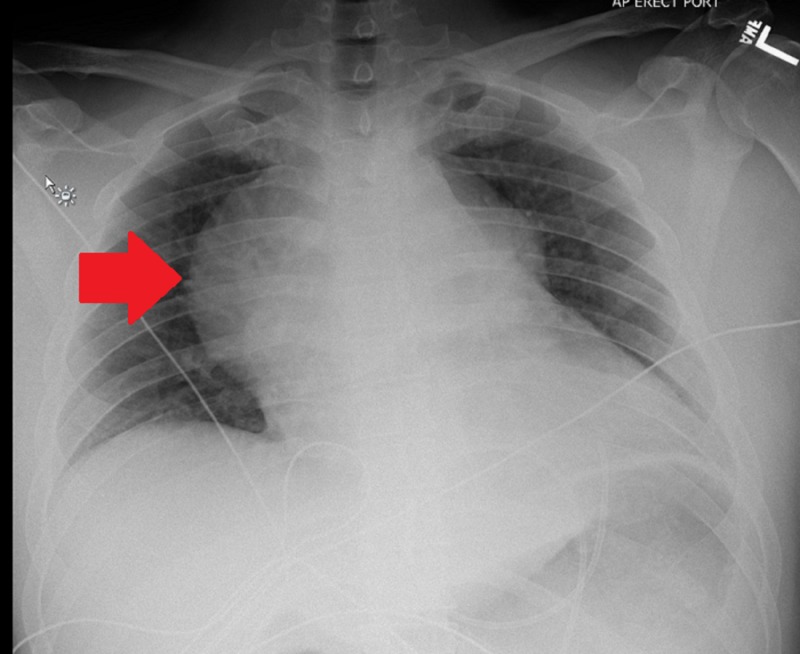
Chest X-ray showing smooth protuberance in the right perihilar region
